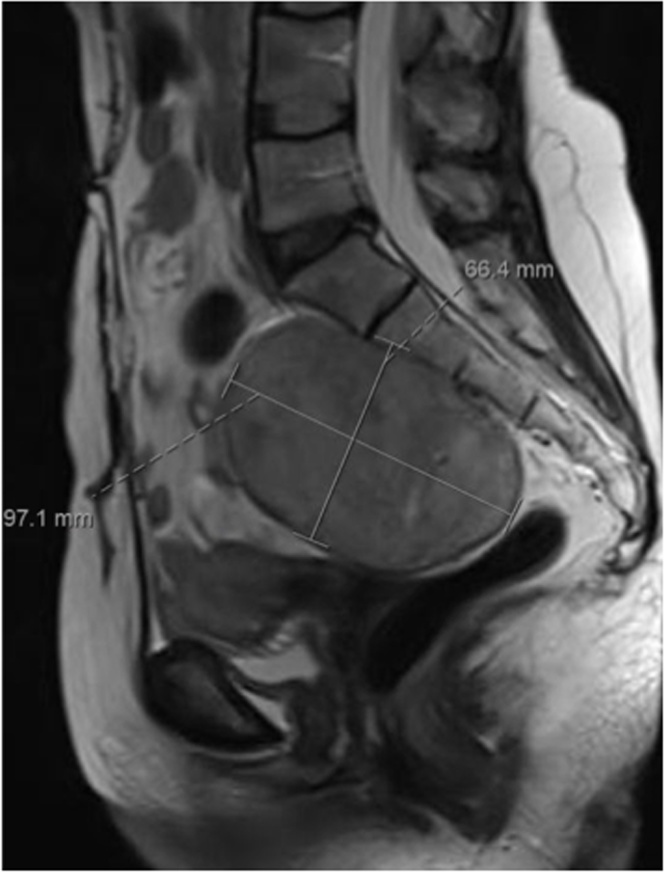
MRI of pelvis with contrast showing a large encapsulated mass located at the presacral region from S1 to S3 measures 7.5 × 6.6 × 9.7 cm in transverse, AP, and long axis respectively.
